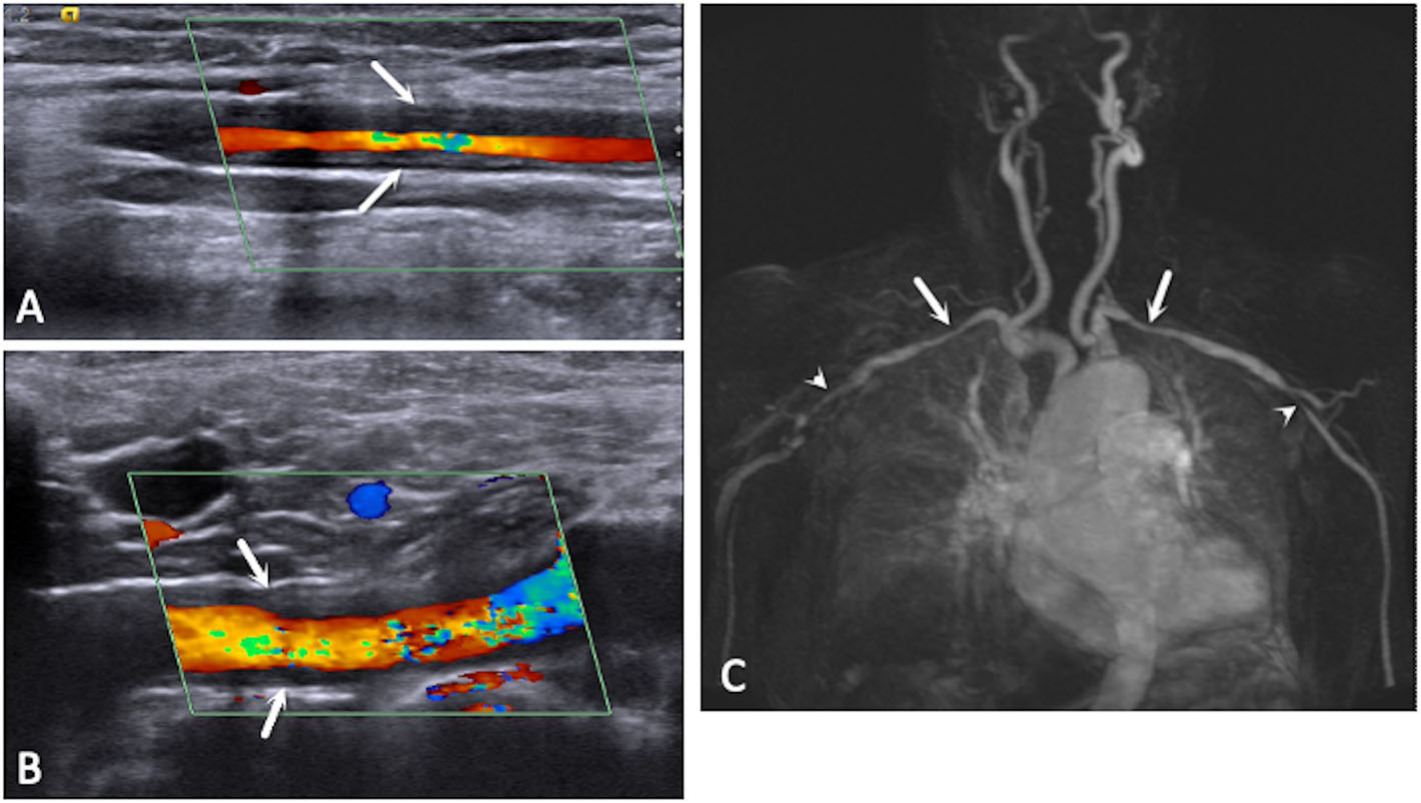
Ultrasound images revealing homogeneous hypoechoic increased intima-media thickness of the axillary (a) and subclavian (b) arteries (white arrows). Magnetic resonance angiography (MRA) (c) of the same patient revealing large vessel involvement, with stenosis of both subclavian arteries (white arrows) and axillary arteries (arrowheads)
